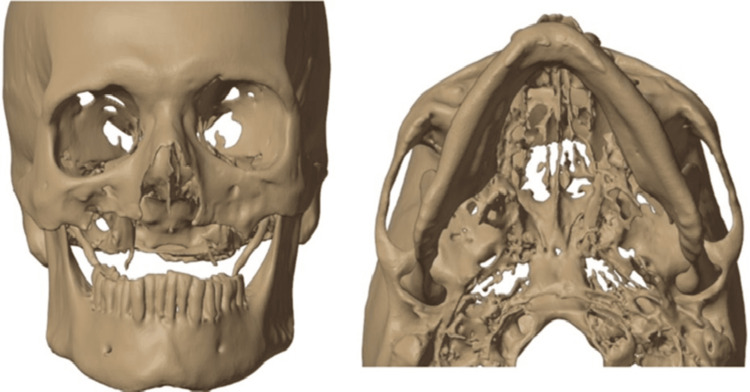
3D model of the skull 3D model of the skull obtained using CT 3D: three-dimensional; CT: computed tomography
